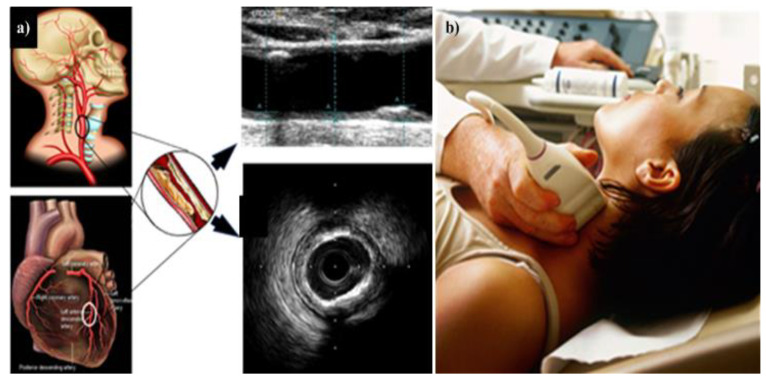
(a) The carotid artery is employed as a proxy for coronary artery disease. (b) Imaging gadget with a linear ultrasound probe scanning the carotid artery. (Courtesy of AtheroPoint, Roseville, CA, USA; produced with permission).
